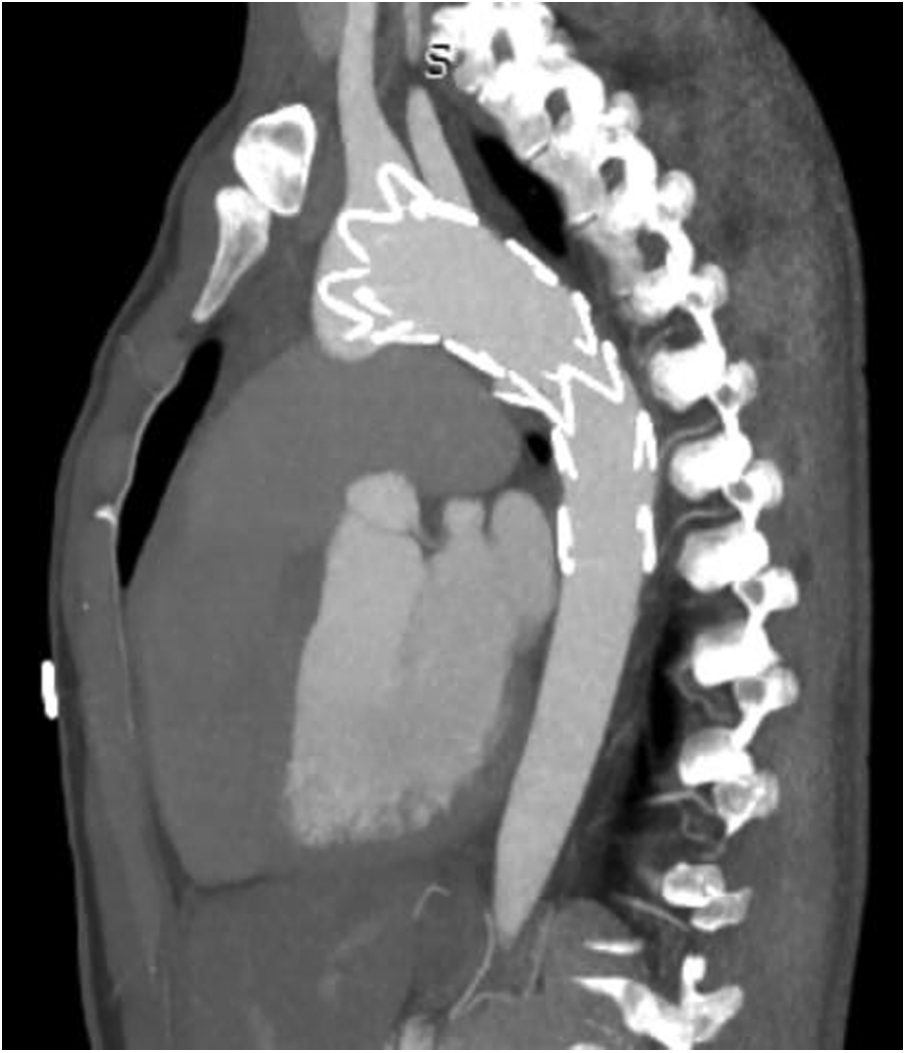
CT angiography after one month showed no endoleak with complete occlusion of PDA shunt. CT, computed tomography; PDA, patent ductus arteriosus.
